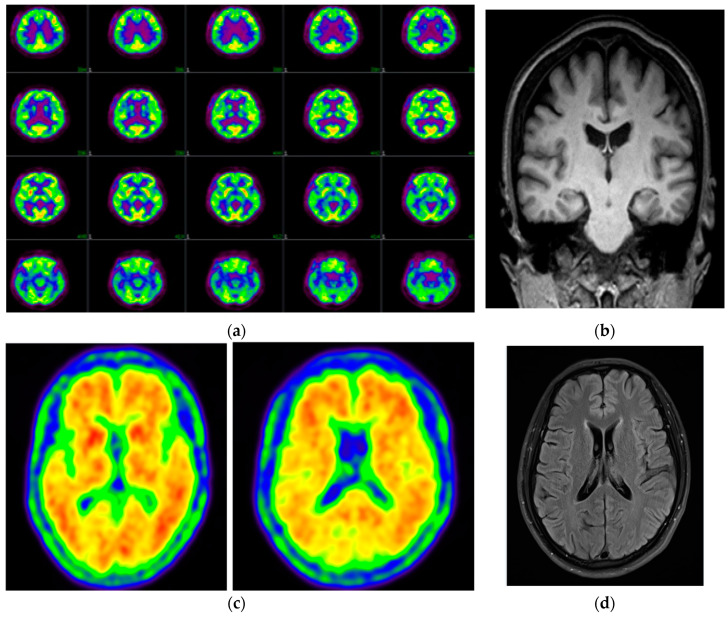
(a) 18F-fludeoxyglucose positron emission tomography (FDG-PET) of patient 1 showed reduced metabolism in different brain areas (bilateral temporal and parietal cortices). (b) Magnetic resonance imaging (MRI) imaging data of patient 1 revealed atrophy in bilateral parietal cortices. (c) PiB-PET data of patient 2 revealed amyloid positivity in several brain regions (bilateral lateral temporal-frontal-parietal areas). (d) MRI imaging data of patient 2 did not reveal any atrophy.
